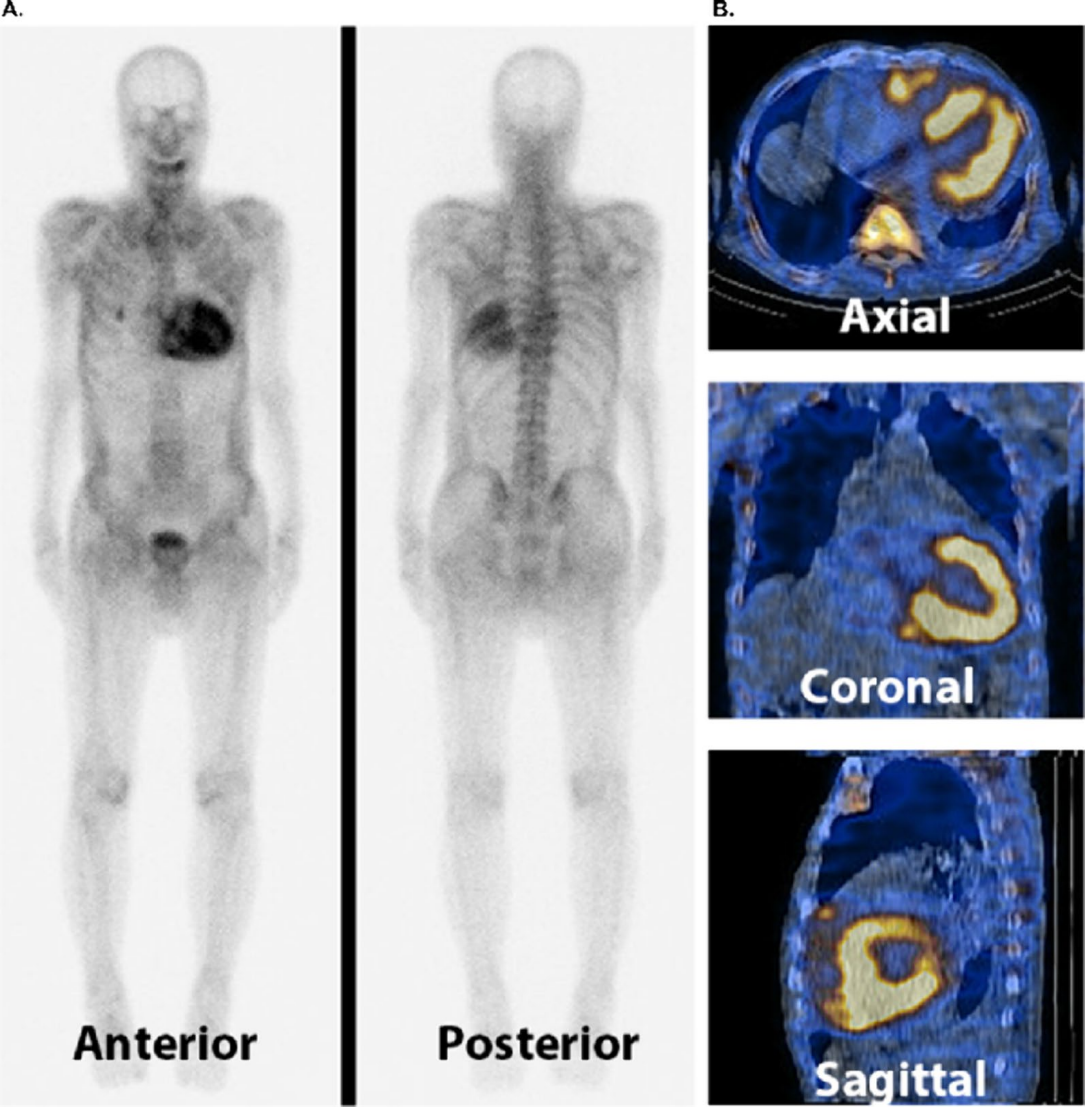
A Technetium pyrophosphate (99mTc-PYP) scintigraphy reveals marked myocardial uptake (Perugini grade 3) of 99mTc-PYP with low skeletal uptake on the whole-body images of the anterior and posterior sides. B Single-photon emission computed tomography-computed tomography images of the heart in the axial, coronal, and sagittal planes show increased myocardial uptake
